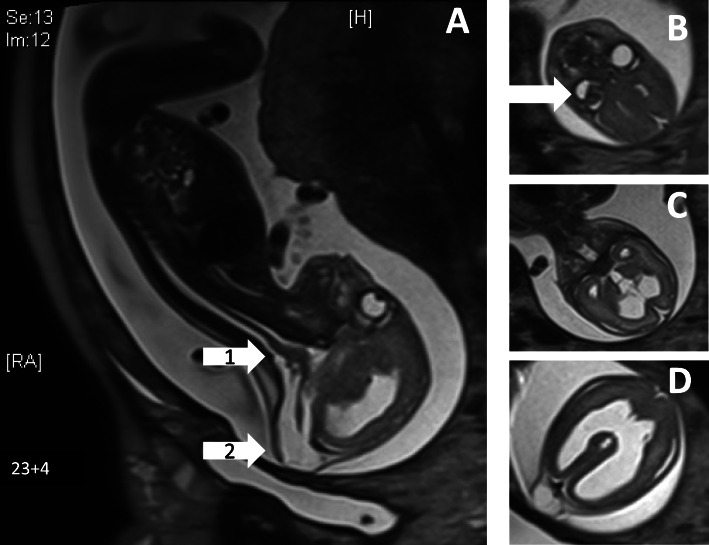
Prenatal MRI scan at 23 + 4 weeks of gestation; a hypoplastic cerebellum (white arrow 1), occipital meningocele (white arrow 2); b persistent hypoplastic primary vitreous body (white arrow); c + d internal hydrocephalus
